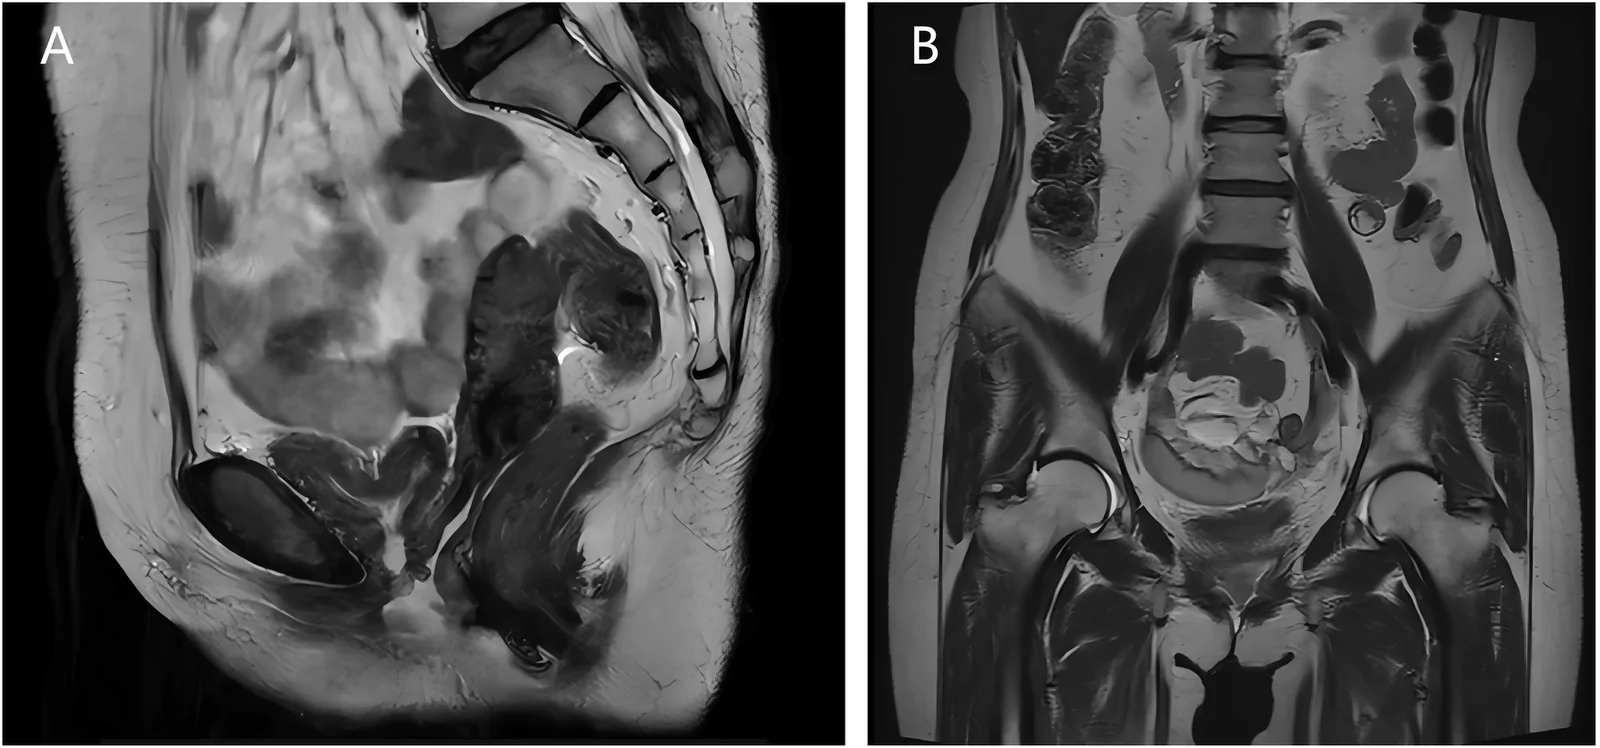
MRI showing no recurrence of the tumor: Sagittal Plane (A), Coronal Plane (B).
